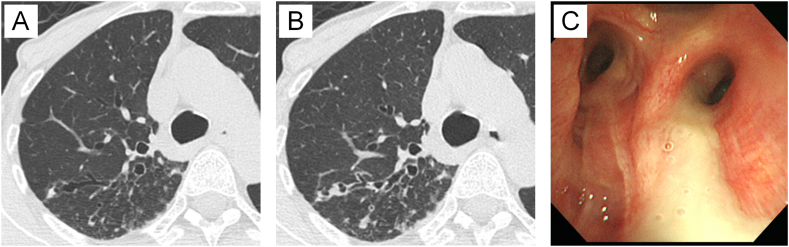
Clinical course of Case 8. Compared to nine months prior [A], chest computed tomography (CT) at the first detection of *Scedosporium apiospermum* showed worsened infiltration and thickening of the bronchial walls [B]. Bronchoscopy revealed a large amount of yellow sputum from right B2 bronchus [C], which was identified as *Scedosporium apiospermum*.
